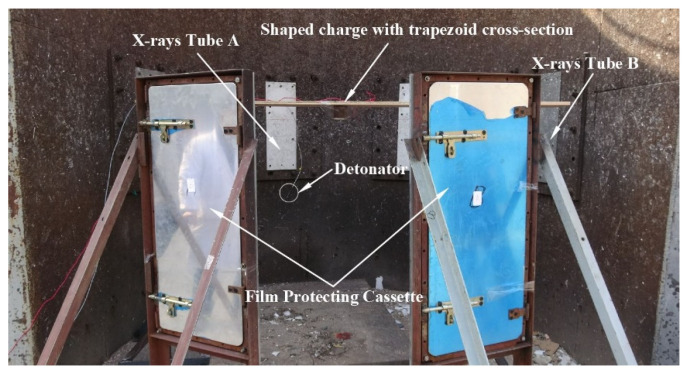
Experimental setup for the X-ray test.
